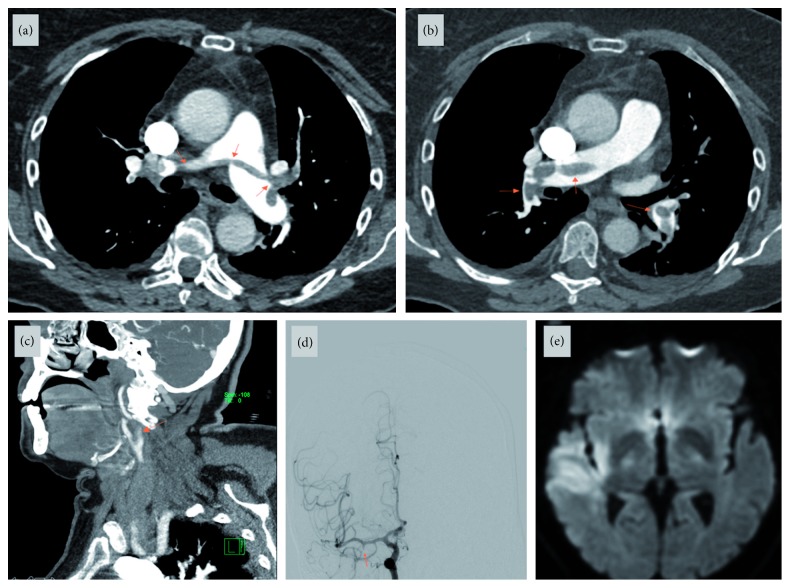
(a, b) Computerized tomography angiography of the chest (axial images) showing “saddle” shaped thrombus in the pulmonary artery bifurcation extending into both trunks (arrows); (c) computerized tomography angiography of the neck (sagittal view) showing right common carotid artery bifurcation showing “saddle-shaped” thrombus in the external and internal carotid arteries (arrow); (d) digital subtraction angiography with selective right internal carotid artery injection showing middle cerebral artery inferior division occlusion (arrow); (e) magnetic resonance imaging of the brain diffusion-weighted imaging axial section showing right frontotemporal area infarction corresponding to the middle cerebral artery inferior branch occlusion.
